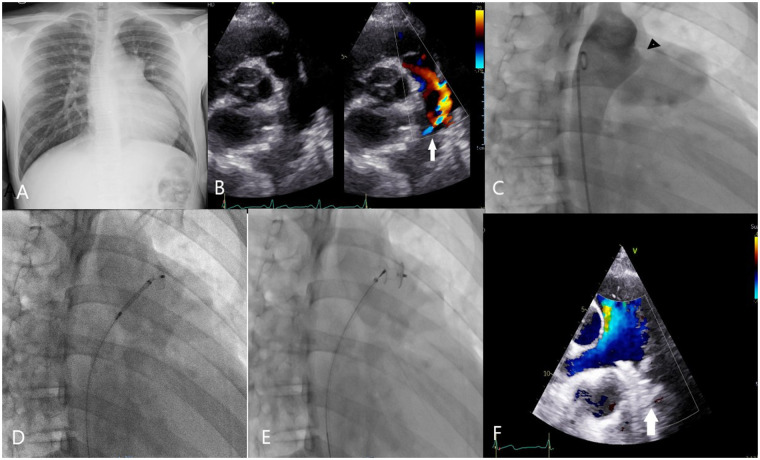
Percutaneous closure of retrograde patent ductus arteriosus with a KONAR-MF™ 6/4. (A) A chest X-ray prior to percutaneous closure of PDA. (B) TTE where we visualize the PDA (white arrow). (C) Aortography in LAO 90°/0° projection showing PDA type E (black arrow). (D) Deploying the first disc of the KONAR-MF™ device. (E) Deploying the second disc of the KONAR-MF™ device prior to delivery. (F) Echocardiography after immediate percutaneous closure of PDA.
